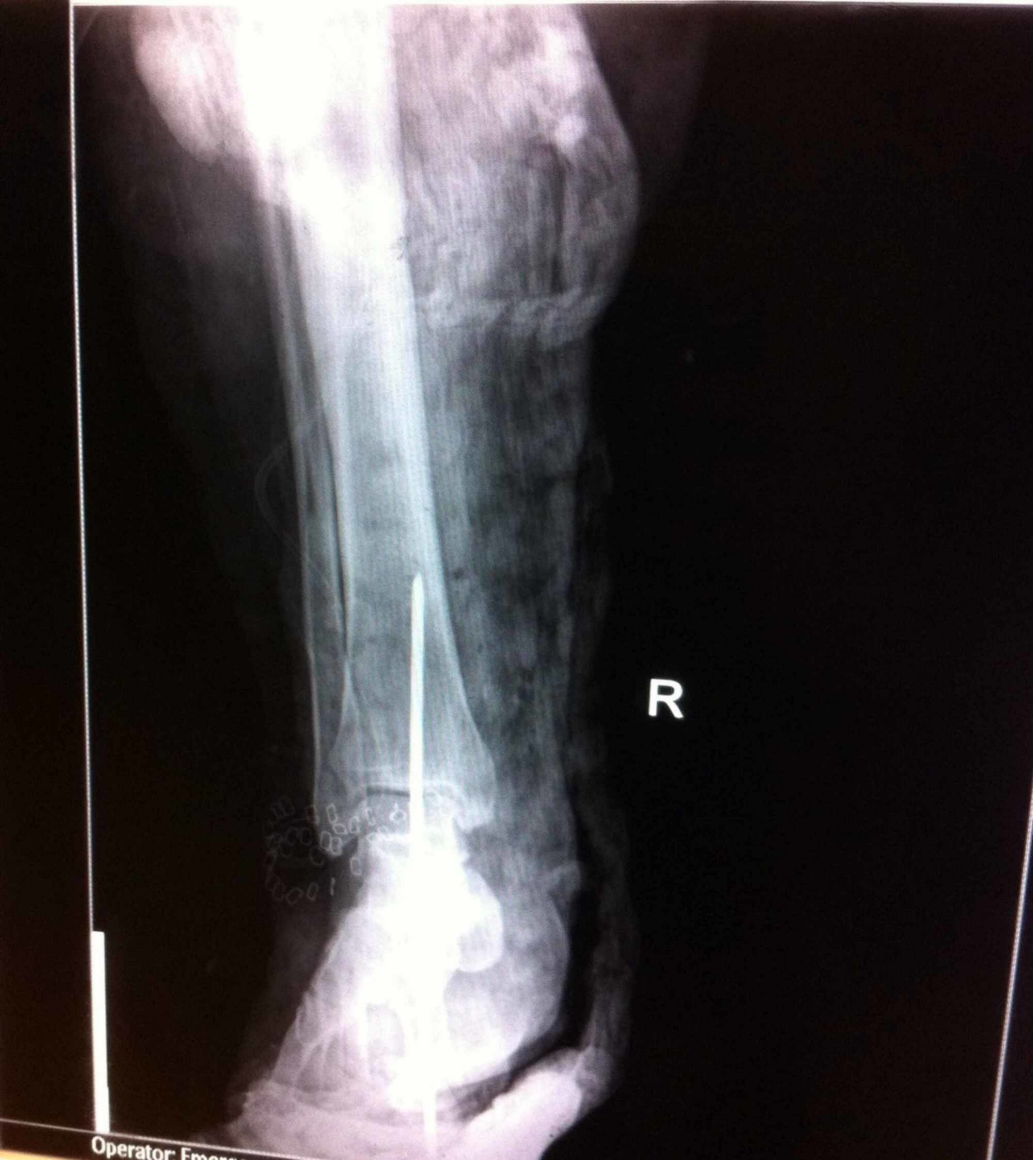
Postoperative antero-posterior radiograph of the right ankle demonstrating proper reduction of the talar dislocation and stabilization by the use of a Steinmann pin
